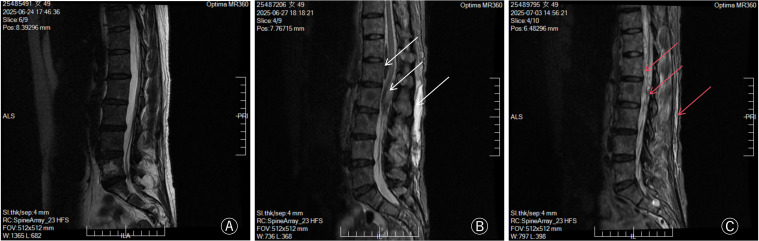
Serial T2-weighted MRI of the lumbar spine. (A) Preoperative sagittal MRI showed posterior disc bulging at L3–L4 and L4–L5. (B) MRI obtained after neurological deterioration showed mixed-signal material within the spinal canal from T12 to S2, with compression and edema of the conus medullaris and cauda equina. The MRI appearance did not clearly distinguish among subarachnoid, subdural, or mixed intradural compartments; the lesion is therefore described as a spinal intradural hematoma. (C) Follow-up MRI obtained after left lower-extremity strength improved to 4/5 showed residual streak-like intradural signal abnormalities anterior to the conus medullaris at T12–L1, posterior to the cauda equina at L2–L3, and at L5–S2, with reduced hematoma extent and less compression than on the prior study.
